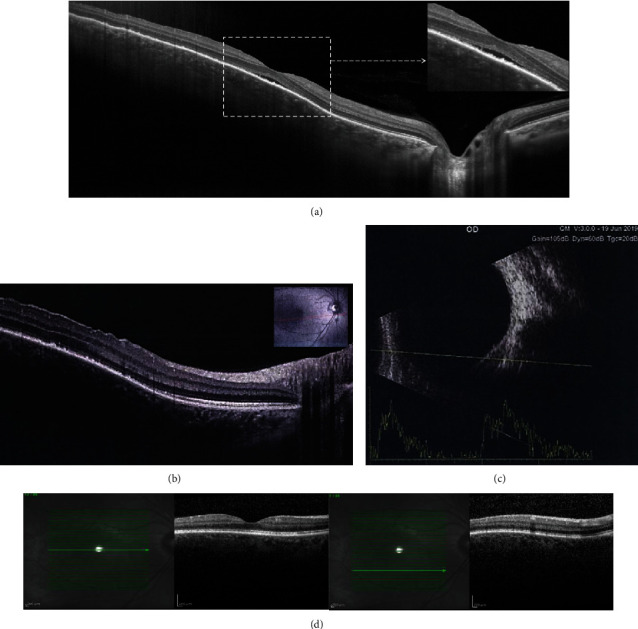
Follow-up examinations. Optical coherence tomography two weeks after initial presentation showed improvement of subretinal fluid, decreasing the size of the choroidal mass, disruption of the ellipsoid zone, and accumulations of hyperreflective material of variable size and shape under the fovea (a) and the corresponding retina over the choroidal mass (b). Ultrasonography two weeks after the initial presentation revealed decreasing the size of choroidal mass (c). OCT one month after initial presentation showed near-complete resolution of choroidal mass and SRF, EZ restoration, and disappearance of hyperreflective materials over RPE and outer retina.
